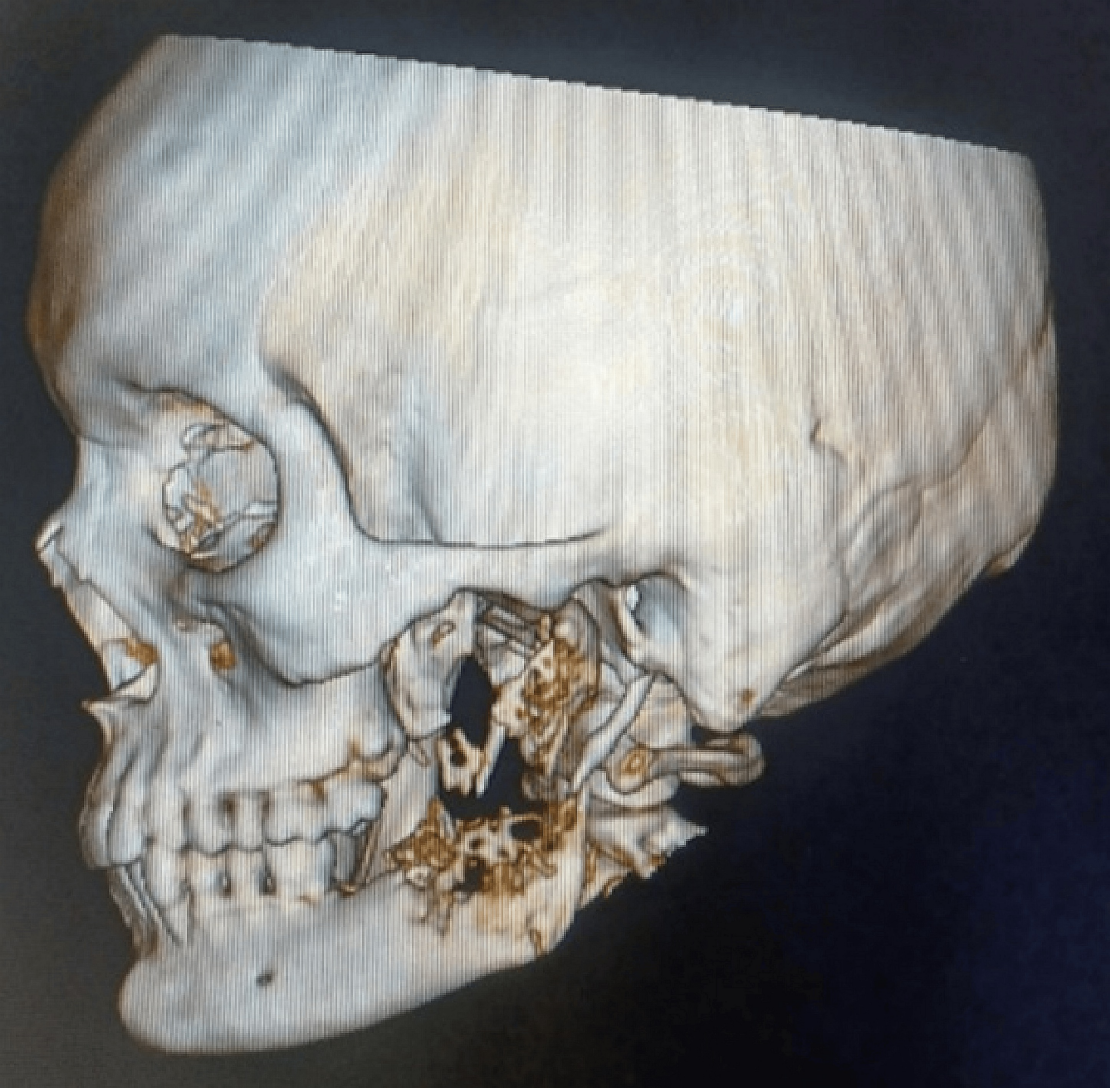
3D CT face showing the extent of the lesion
